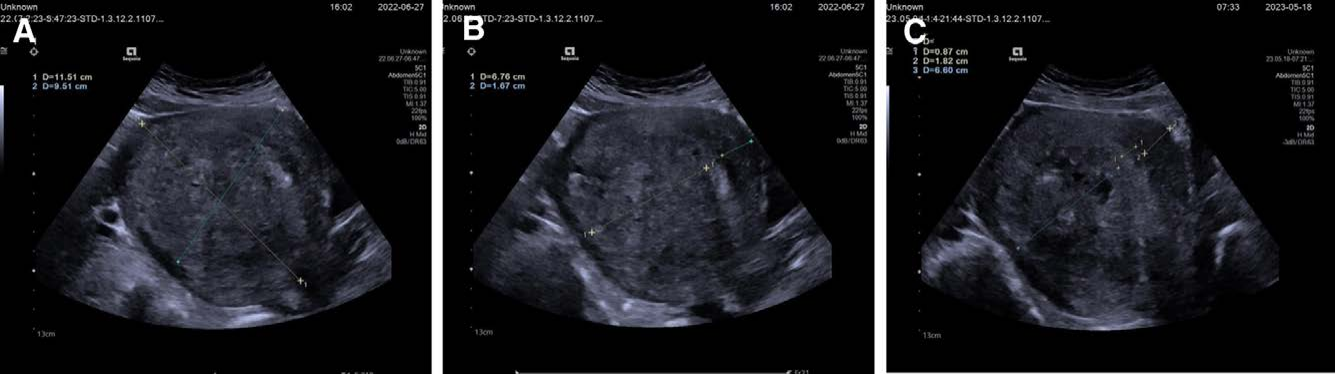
(A–C) Gynecological ultrasound showed uterus size 11.51 × 9.51 cm (A). Pre-MWA uterine wall thickness: anterior wall 1.67 cm, posterior wall 6.67 cm (B). Post-MWA 11 months: anterior wall 1.82 cm, posterior wall 6.60 cm (C).
